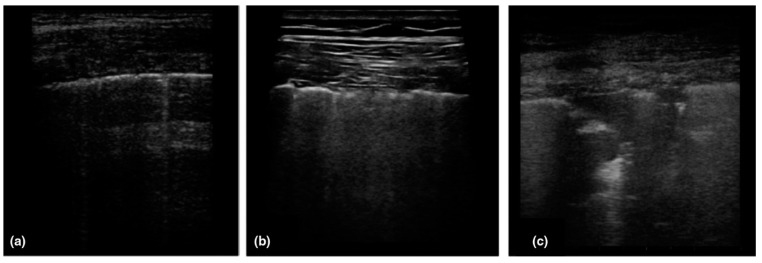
Patterns of COVID-19 at chest ultrasound. (a) Early bilateral multifocal areas of interstitial syndrome, (b) Interstitial pneumonia characterized by interstitial syndrome with B lines and preserved sliding sign, (c) Advanced, and organized pneumonia with interstitial syndrome associated with multiple subpleural consolidations and reduced sliding sign. Reproduced with permission. Ref. [1] Journal of Internal Medicine © 2022 John Wiley and Sons, Inc.
